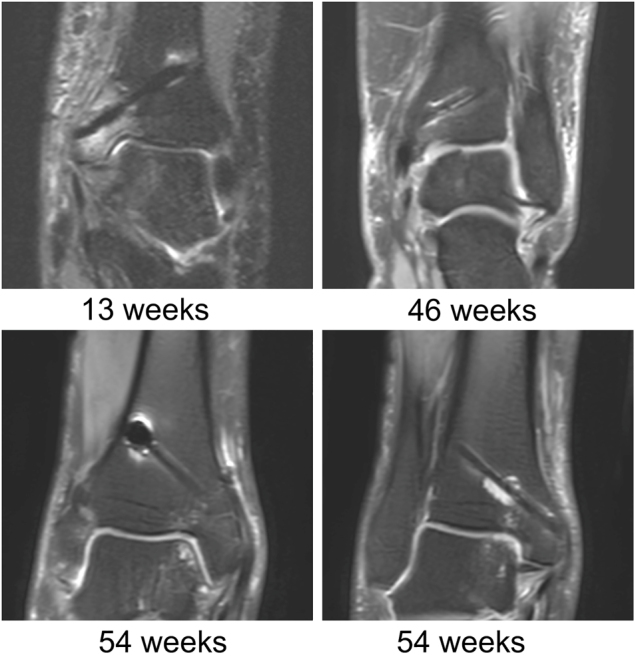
Examples of MRI findings in proton density weighted (PDw) sequences for different time points and patients: bone marrow edema in the medial malleolus (13 weeks postoperatively), visibility of a hyperintense line in the screw center (46 weeks postoperatively), gas accumulation at the screw tip (54 weeks postoperatively), and liquid collection around the screw (54 weeks postoperatively).
